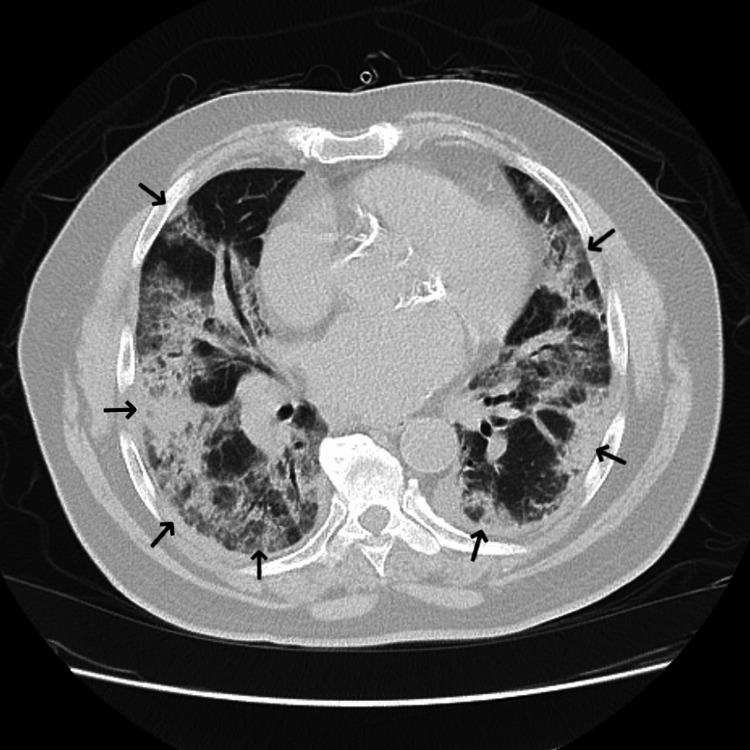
An axial slice of the computed tomography pulmonary angiogram on day 1 of admission showing diffuse bilateral infiltrates
